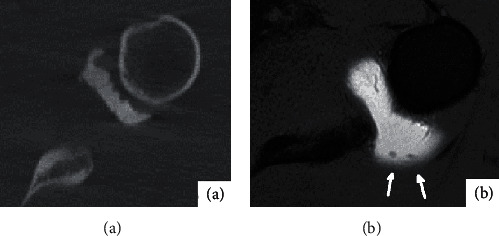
Male, 19 years old, with anterior dislocation of the left shoulder for 2 months. (a) is the bone window image of CTA transverse axis of the left shoulder. No obvious free cartilage tablets were found in the joint capsule. (b) shows the T1WI-FS image of the left shoulder in the horizontal axis of MRA. White arrows show two free small cartilage tablets in the joint capsule.
